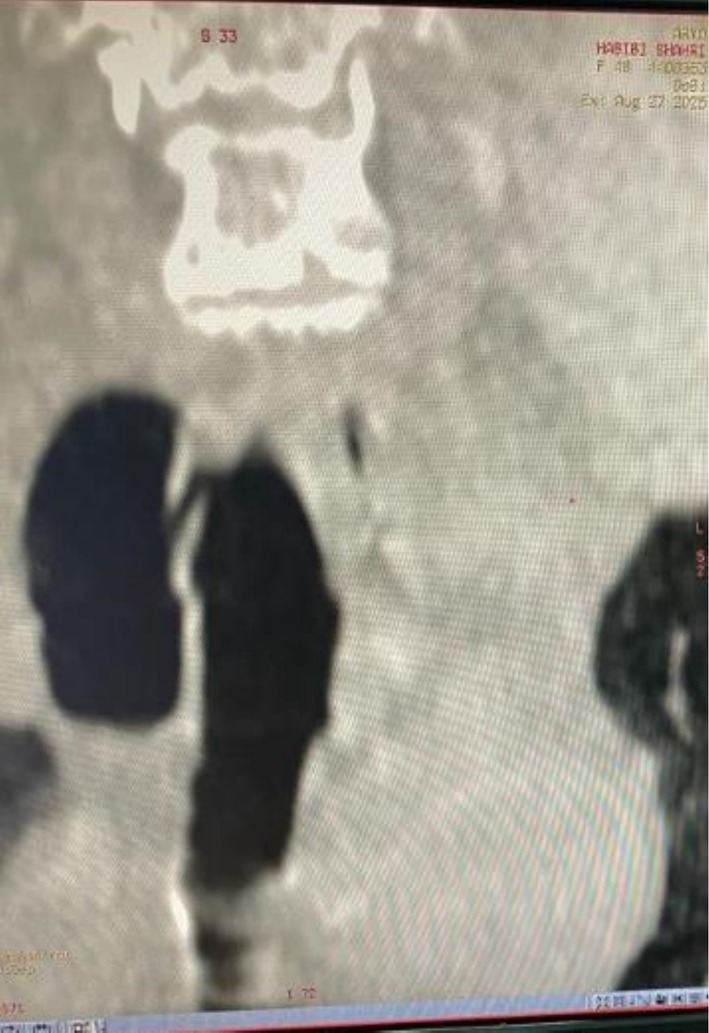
Coronal reformatted CT image demonstrates the tracheal diverticulum in the right posterolateral position. The image shows the cranio‐caudal extent of the lesion and its relationship to surrounding structures.
